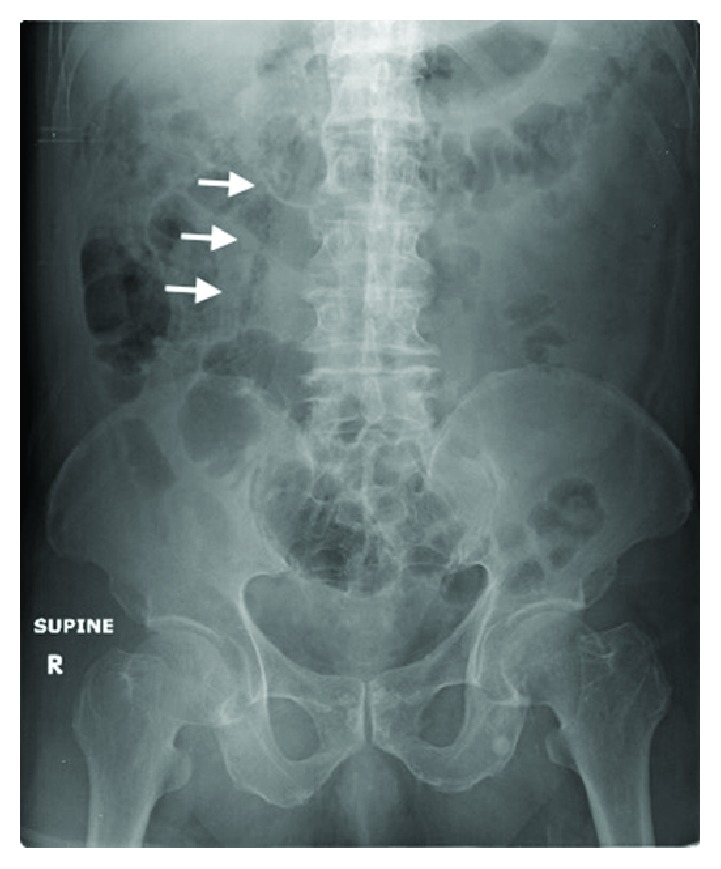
Abdominal X-ray showing lucency outlining the right crus of the diaphragm and extending along the right psoas muscle.
